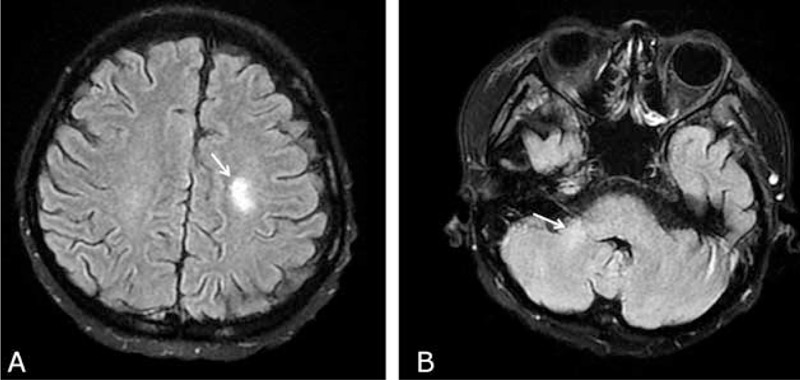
Brain magnetic resonance imaging on day +72 posttransplant showing T2-hyperintense lesions (arrows) in the (A) deeper site of left frontal lobe and in (B) right middle cerebellar peduncle.
